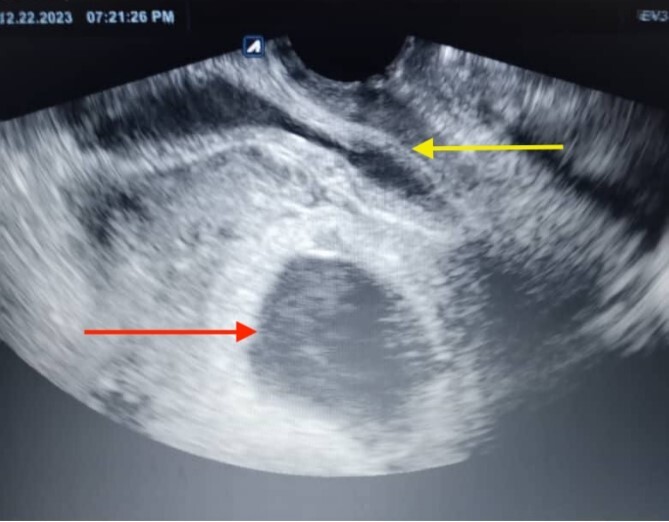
The ultrasonographic imaging conducted the day following the patient’s hospital admission revealed a ruptured gestational sac (the lower arrow), which was apparent outside the uterus. The uterine mass was visible on one side (the upper arrow), and the gestational mass was on the other side.
